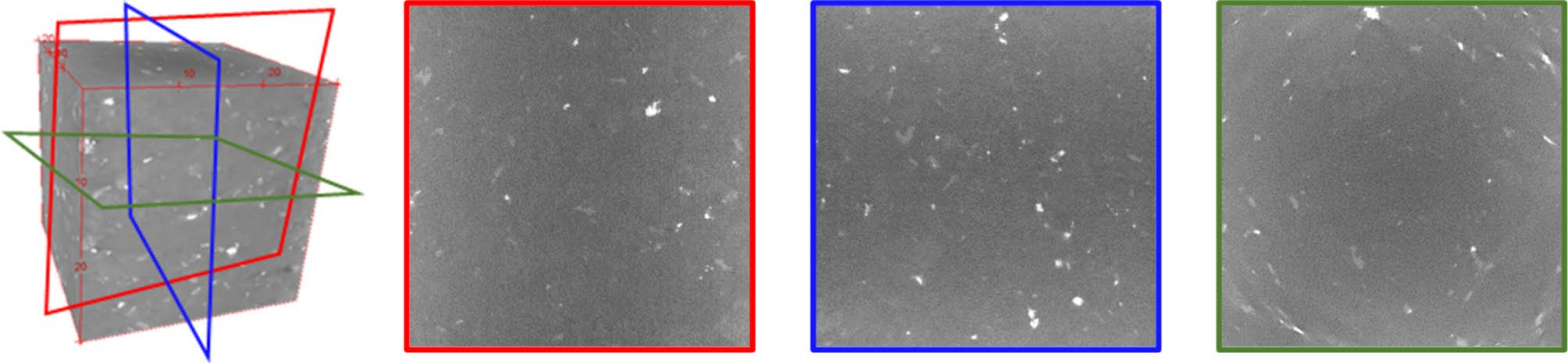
Examples of X-ray computed tomography images corresponding to each cleavage plane: Rift (red), Grain (blue), and Hardway (green).
